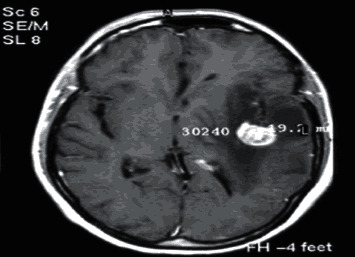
Brain tumor on CT-scan [12].
